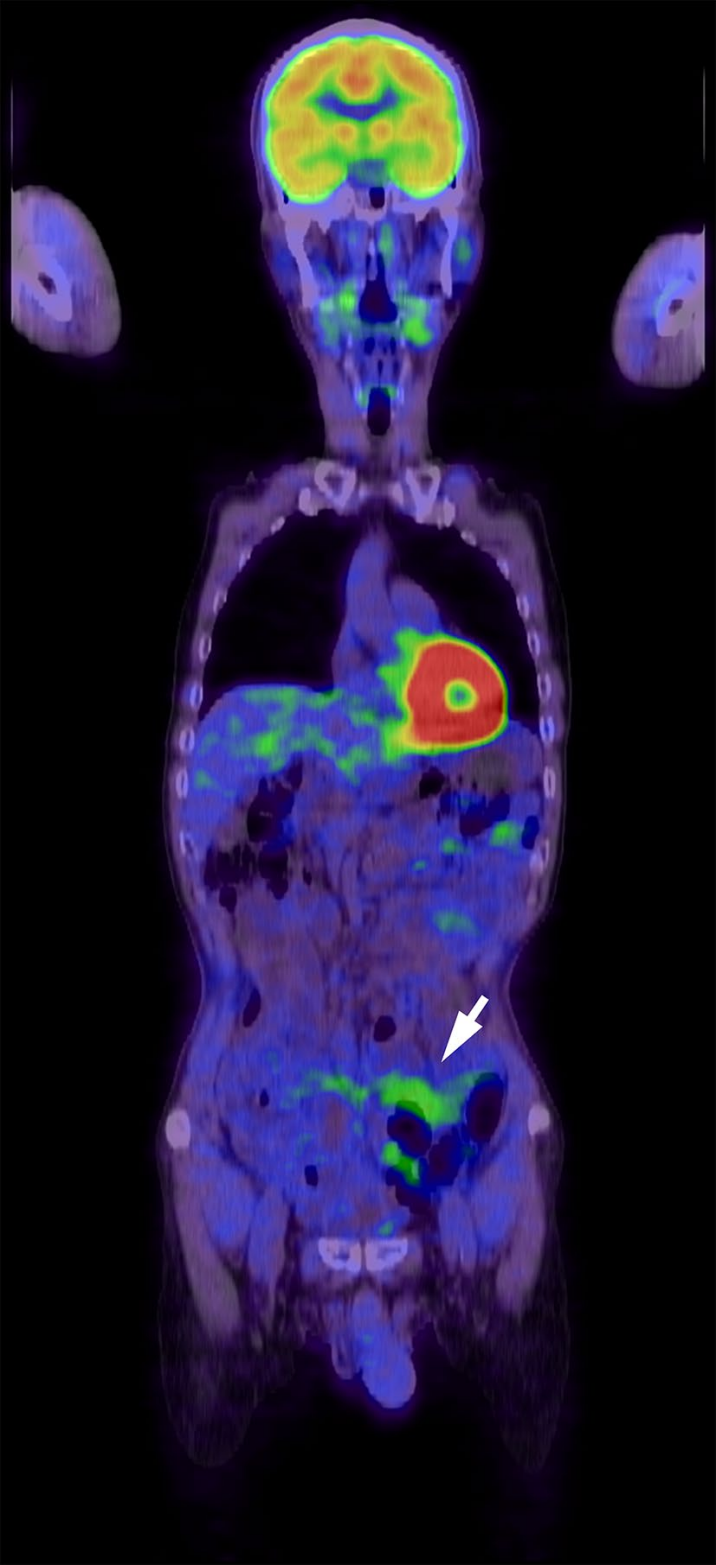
Whole-body FDG-PET imaging. Abnormal FDG uptake was not detected by PET in the patient’s body, including GI tract and lymph nodes. Arrow points to the enlarged small intestine
